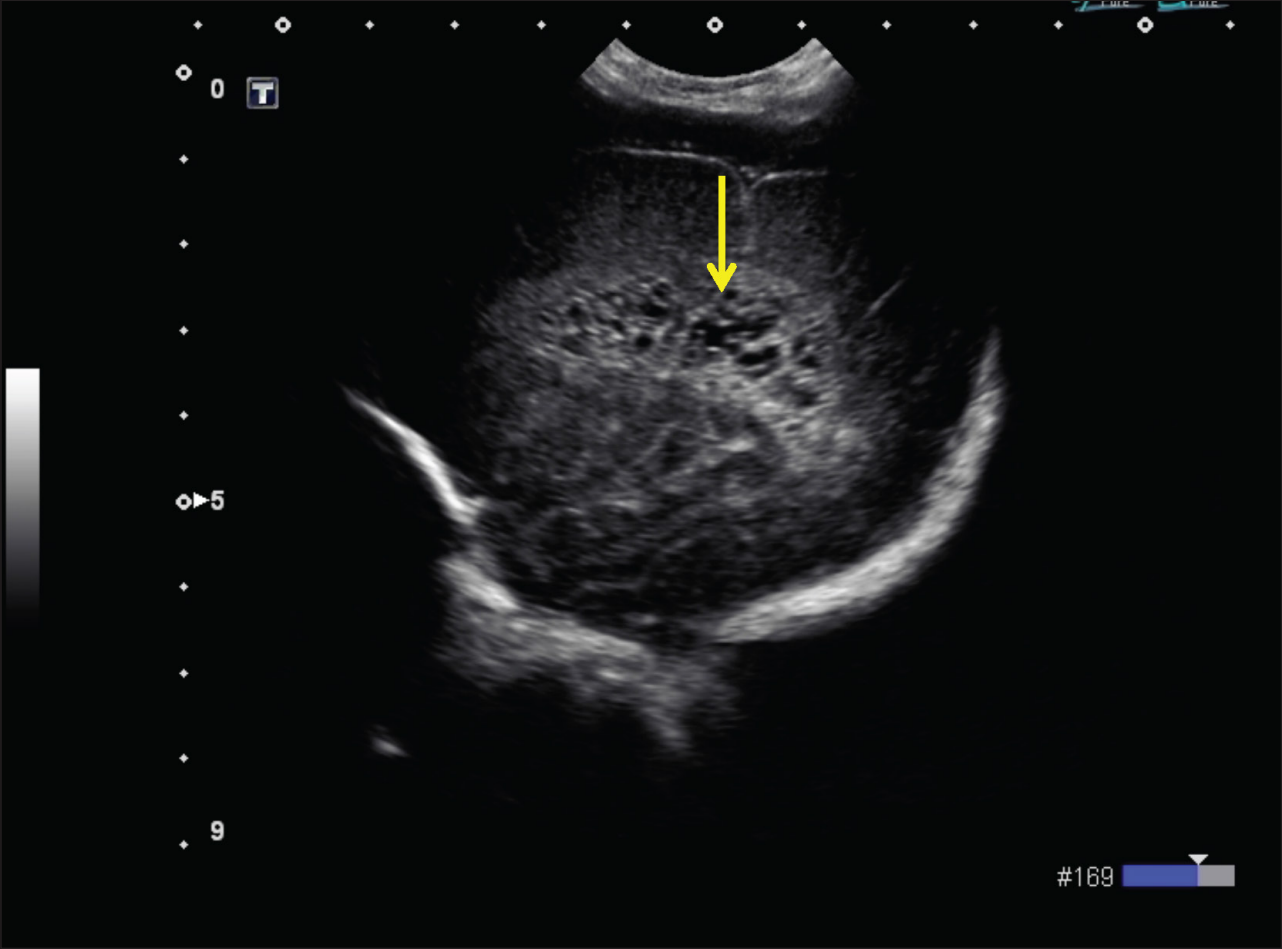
Transcranial ultrasound, 8 weeks post-partum, parasagittal plane. Extensive periventricular cysts (arrow).
